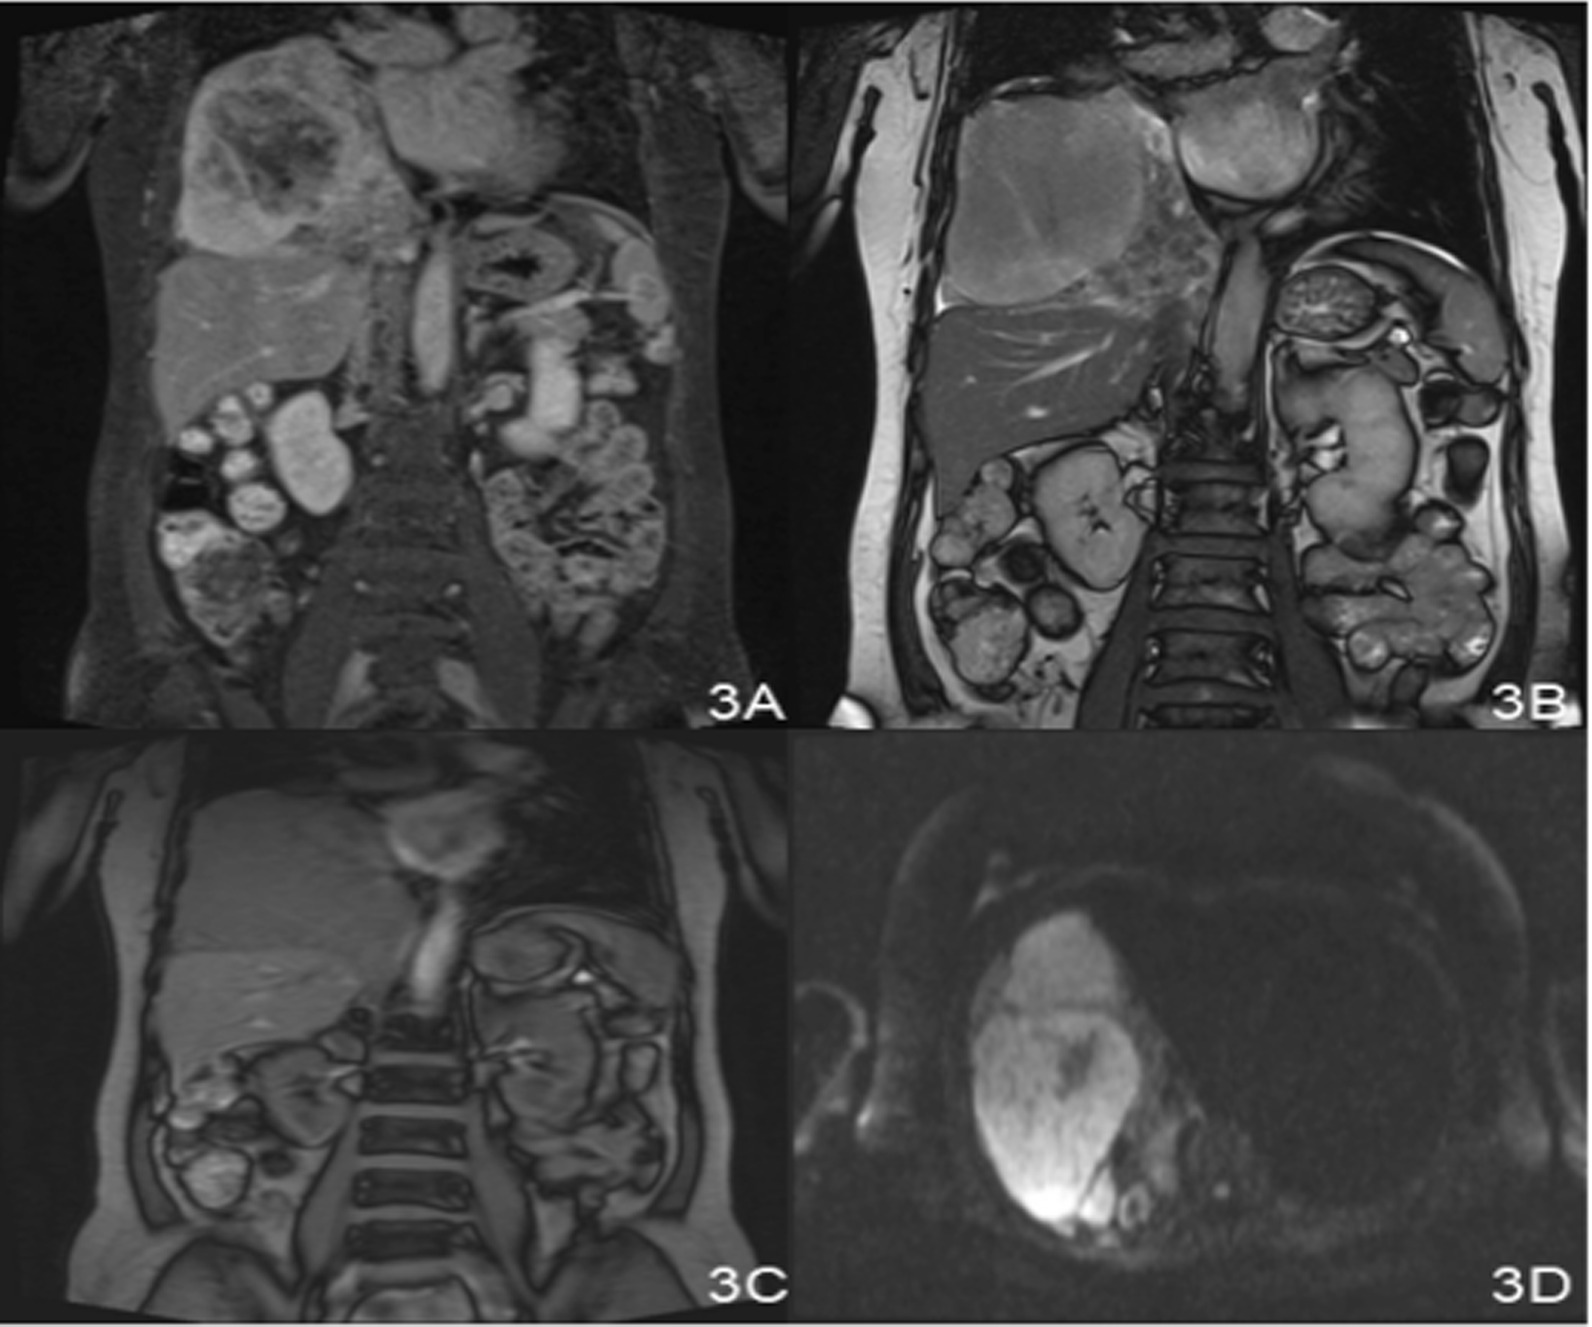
A, B, C Coronal view of chest MRI examination showing the maximum diameter of the mass located in the chest cavity; the right lung lobe is compressed, and the mass has a distinct border with the underlying liver tissue. D The mass showing a significant high signal on diffusion weighted imaging (DWI)
